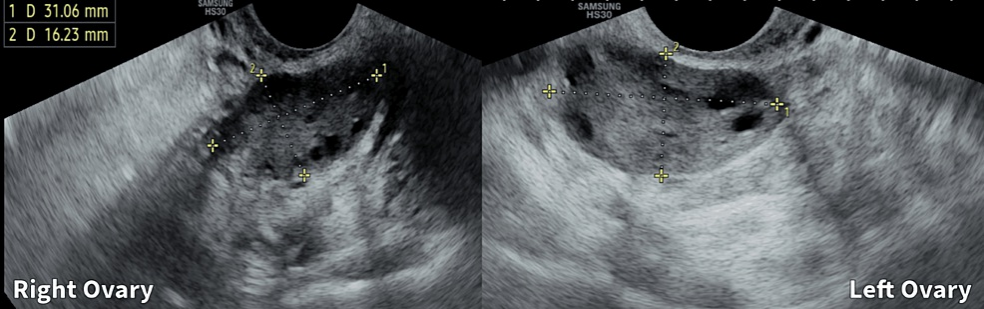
Post-intervention transvaginal sonography image of the patient's right and left ovaries on day 2 of menstrual cycle.
